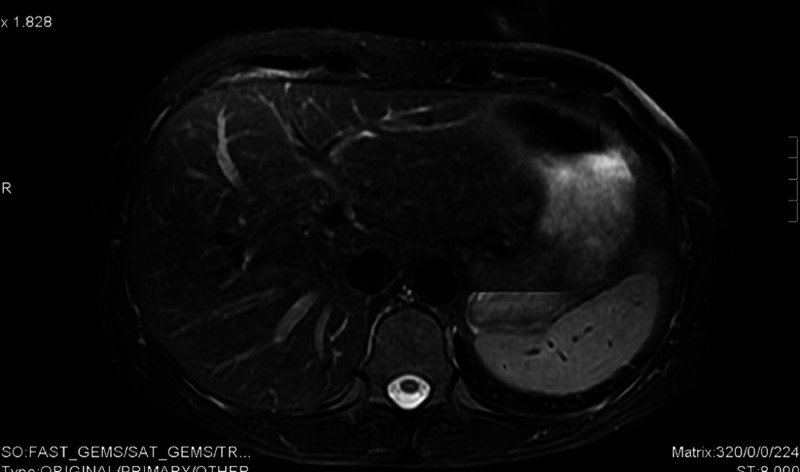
Patient 3: Magnetic resonance imaging (MRI) liver reveals resolution of liver metastasis post treatment
